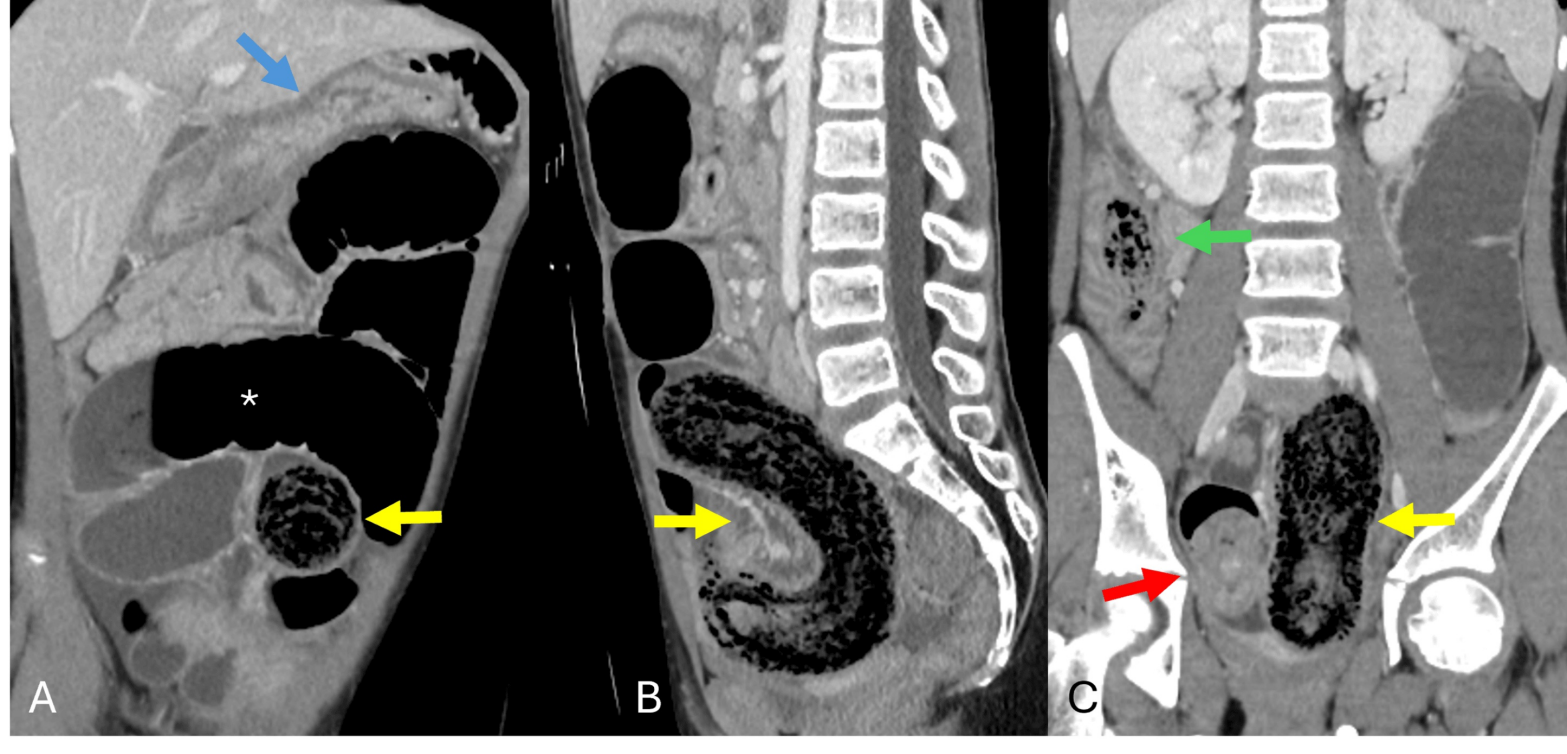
Contrast-enhanced CT images of the abdomen and pelvis demonstrating distal migration of the trichobezoar into the distal ileum with extension into the ascending colon. (A) The coronal image shows an empty stomach (blue arrow) with interval migration of the trichobezoar to the distal ileum (yellow arrow) and associated upstream small bowel dilation (*) consistent with small bowel obstruction. (B) The sagittal image demonstrates the stomach-shaped trichobezoar (yellow arrow) within and obstructing the distal ileum. (C) The coronal image shows the bulk of the trichobezoar (yellow arrow), associated distal ileoileal intussusception (red arrow), and the bezoar “tail” extending into the ascending colon (green arrow).
